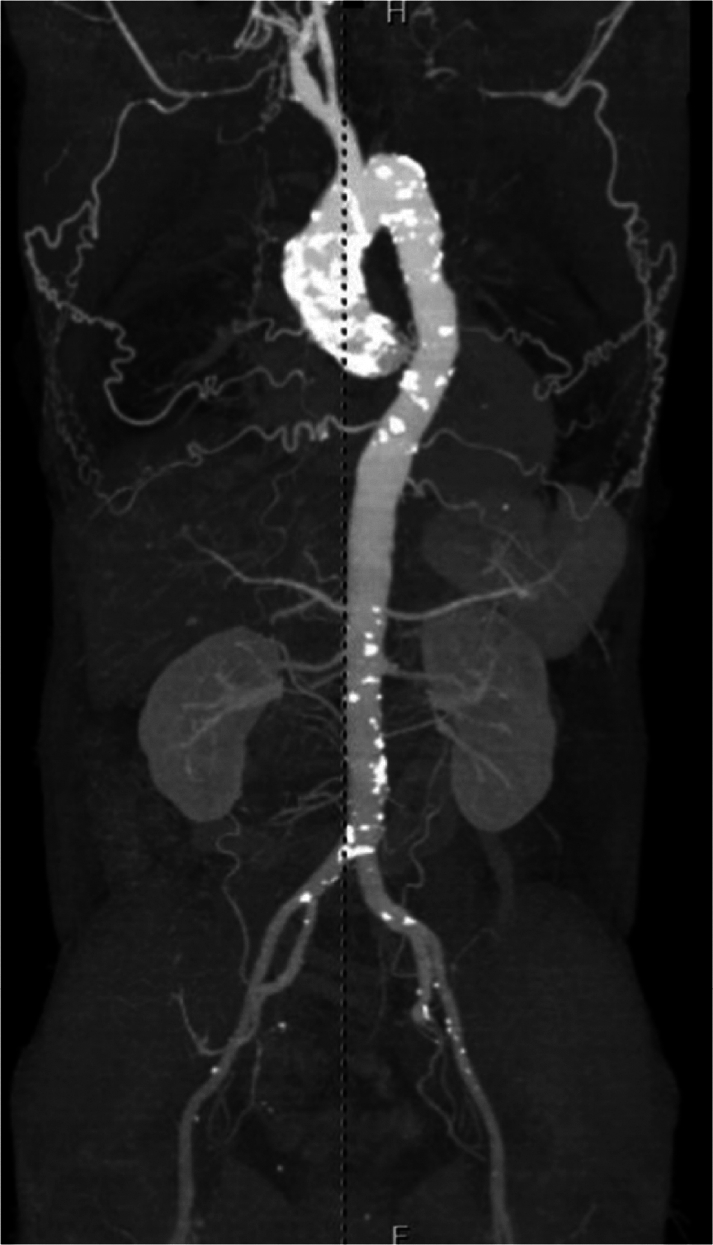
Imaging of Patient 1 Computed tomography revealed circumferential aortic calcification between the aortic root and the descending aorta, left common carotid artery occlusion, and circumferential calcification of both subclavian arteries and the internal thoracic artery.
